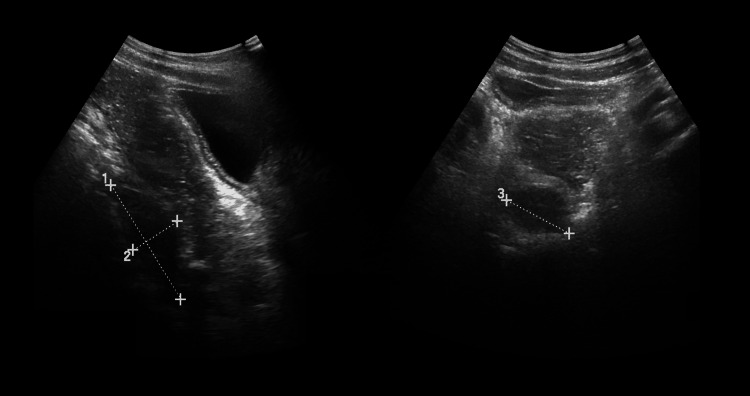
Ultrasound image of the pelvis showing a retrorectal fluid collection measuring approximately 6.5 × 2.5 × 3.4 cm (30.45 mL) with internal debris. This collection was managed conservatively with intravenous antibiotics and follow-up imaging.
